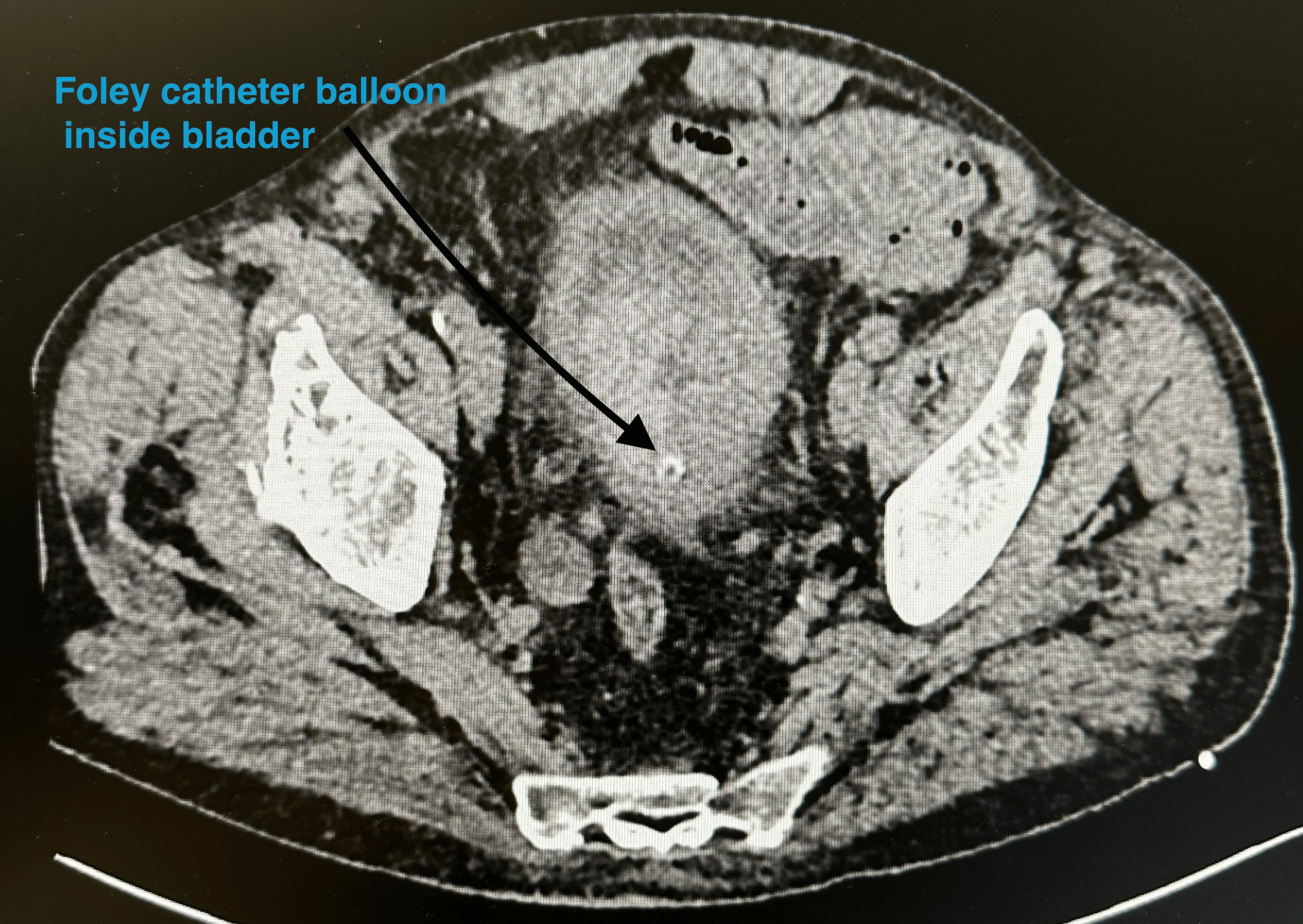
Axial CT scan at the level of the pelvis demonstrating a Foley catheter balloon inside the urinary bladder (black arrow) The bladder is enlarged and contains heterogeneous material suspicious for tumor debris or clot CT: computed tomography
